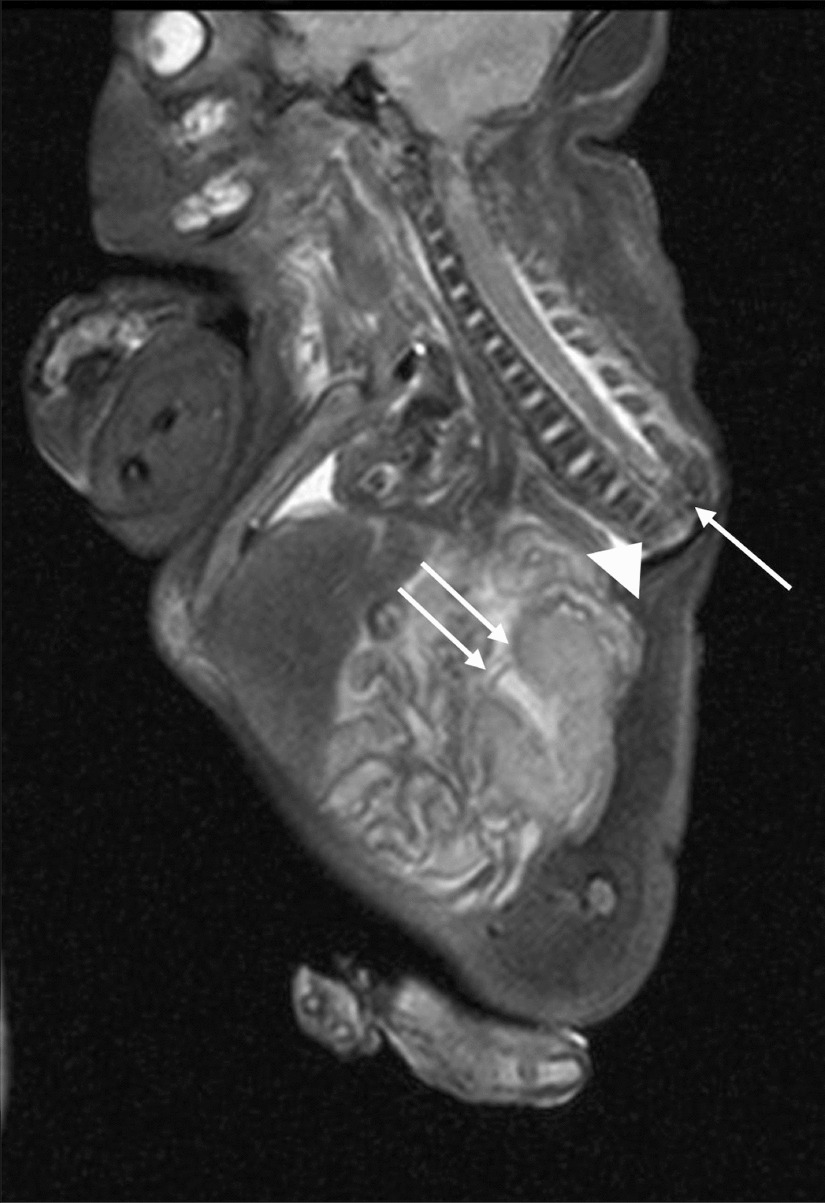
Postmortem MRI autopsy of the stillborn male. T2 WI sequence shows truncated spinal cord (arrow), vertebral column terminating at T7 vertebra (arrow head), and horseshoe kidneys (double arrows)
